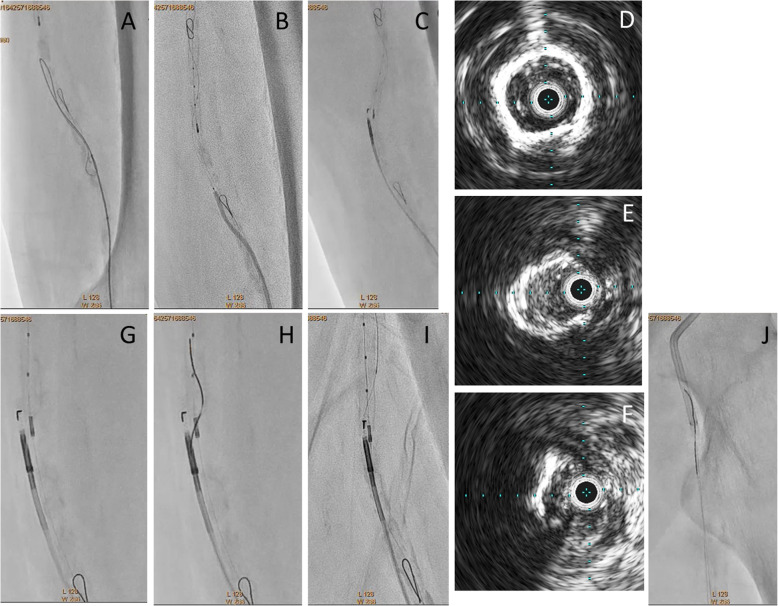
a: Advancement of knuckle-shaped 0.035-in. Radifocus wire. b: Both wires are closed. Antegrade intravascular ultrasound showing that the antegrade wire is in the intraplaque space and the retrograde wire is in the subintimal space. The retrograde route is dilated with a 3.0-mm balloon to enable the advancement of the Outback® Elite catheter (Cordis, Florida, USA). c: The Outback® Elite catheter is advanced retrogradely under intravascular ultrasound guidance from the antegrade direction. d: The IVUS findings of proximal SFA showed 360 degree heavy calcification. e: The IVUS findings showed 180 to 270 degree calcification. f: The IVUS findings from retrograde showed the retrograde IVUS catheter was in the subintimal space, and true lumen was relatively few calcified parts. This was the place where we tried out re-entry from retrograde using Outback® Elite catheter. g: Use of the Outback® Elite catheter. Adjustment of the L marker. h: Adjustment of the T marker. i: Successful re-entry. j: Advancement of the retrograde wire into the antegrade guiding sheath
